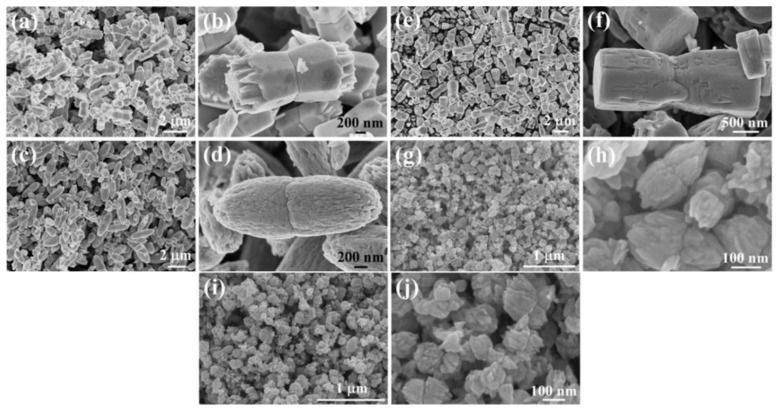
SEM images of ZnO products prepared under different Zn/2-[4-(2-hydroxyethyl)-1-piperazinyl]ethanesulfonic acid molar ratios: 3:8 (a,b); 1:4 (c,d); 1:8 (e,f); 1:20 (g,h); 1:40 (i,j). Products synthesised the by microwave and ultrasonic wave combined method. Reprinted from [844], Copyright (2013), with permission from Elsevier [OR APPLICABLE SOCIETY COPYRIGHT OWNER]. All rights reserved. In order to re-use permission must be obtained from the rightsholder.
